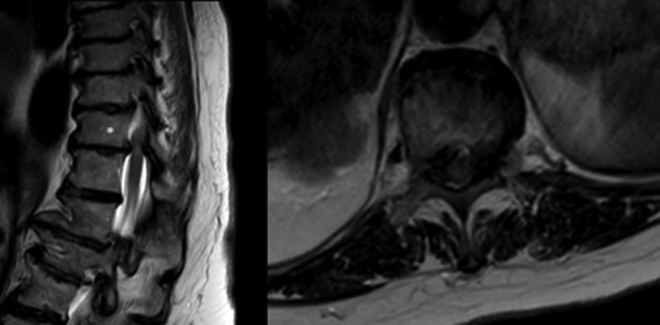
Midsagittal and axial T2 sequence magnetic resonance imaging scans of T11-12 herniated disc (T11 noted with asterisk). Compression was lateral on both sides. Calcification was demonstrated intraoperatively. Interpretation below T11 is obstructed by coronal deformity.
